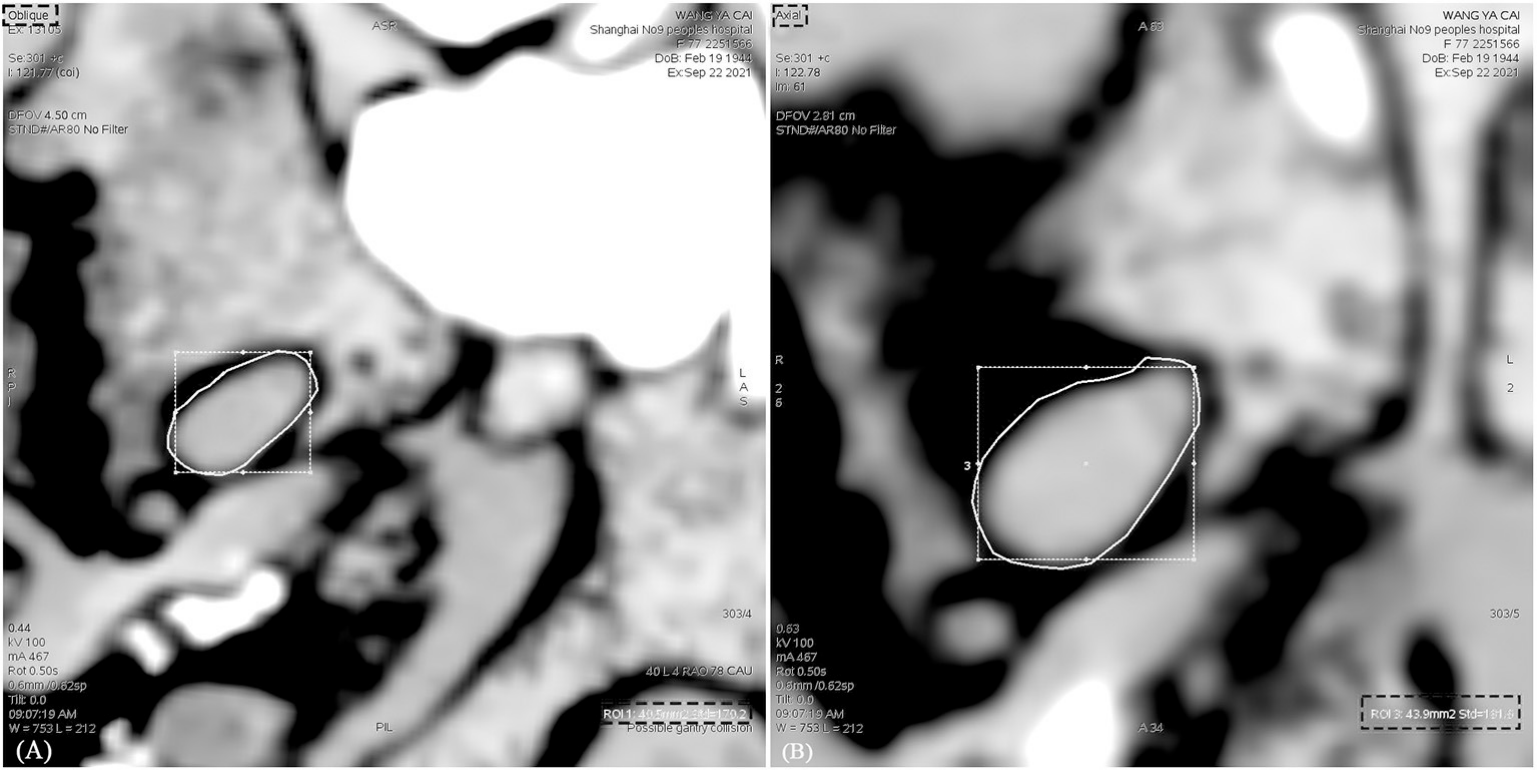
Measurement of the area of the foramen ovale on CT images in different position. (A) The area of the foramen ovale was measured in the oblique position. (B) The area of the foramen ovale was measured in the axial position. The area measured in the axial position is greater than that in the oblique position.
